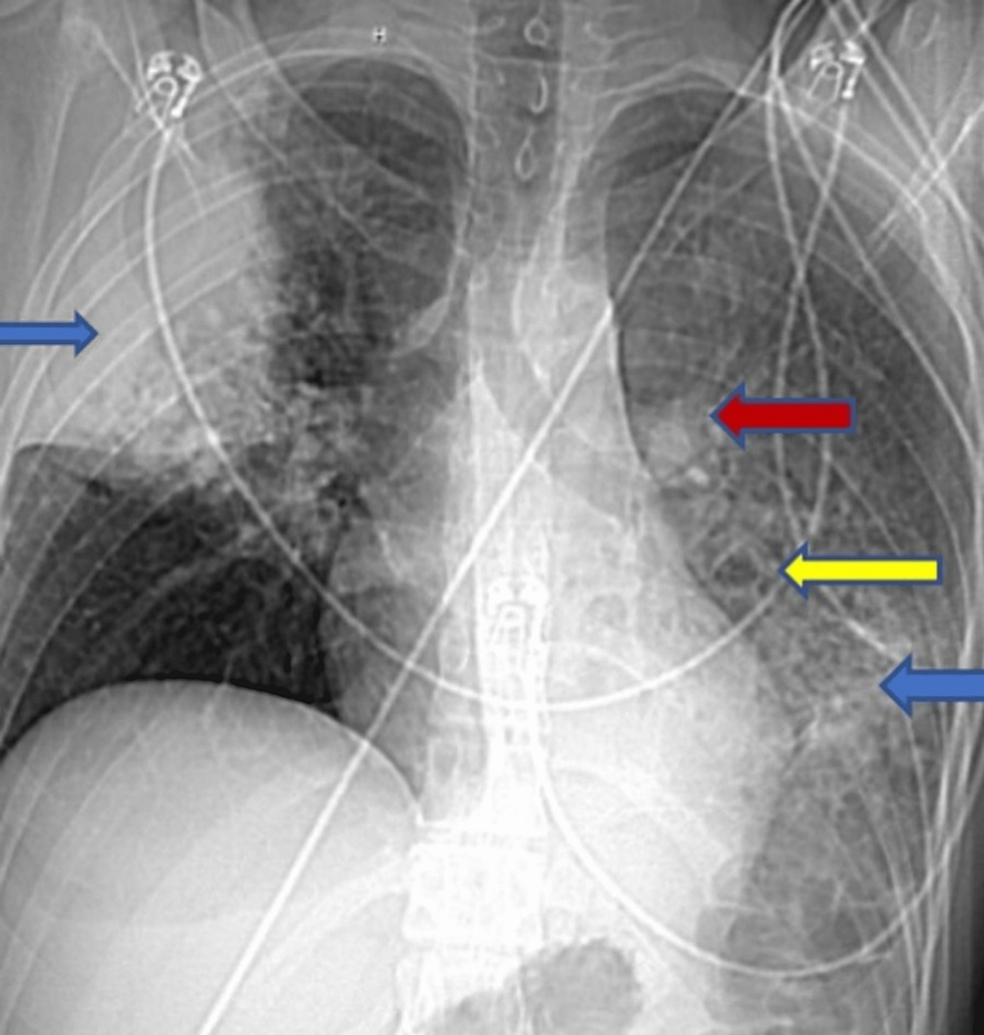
Chest X-ray image The image shows consolidation (blue arrow), nodularity (red arrow), hilar lymph node (yellow arrow).
